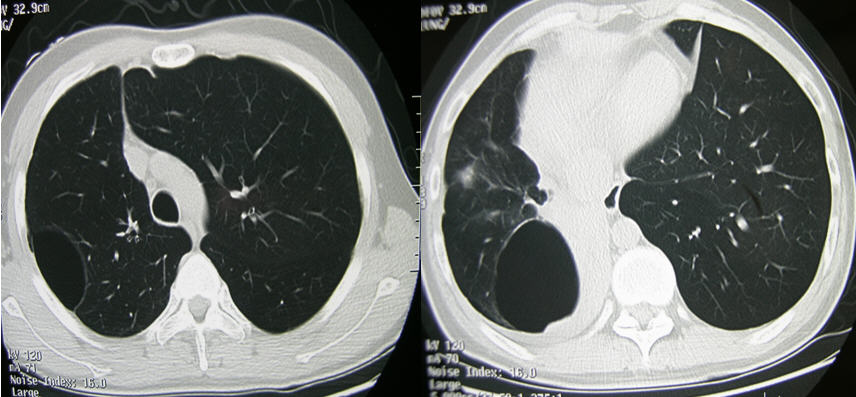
Chest CT shows multiple blebs in right upper lobe and middle lobe, right lower lobe collapse.
